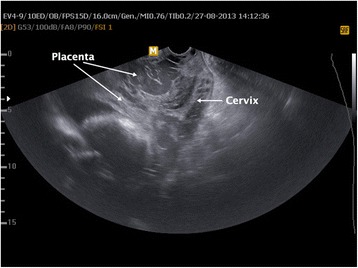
Antepartum transvaginal ultrasonographic survey. Note the tortuous vessels anterior to the lower uterine segment just overneath the cervix
